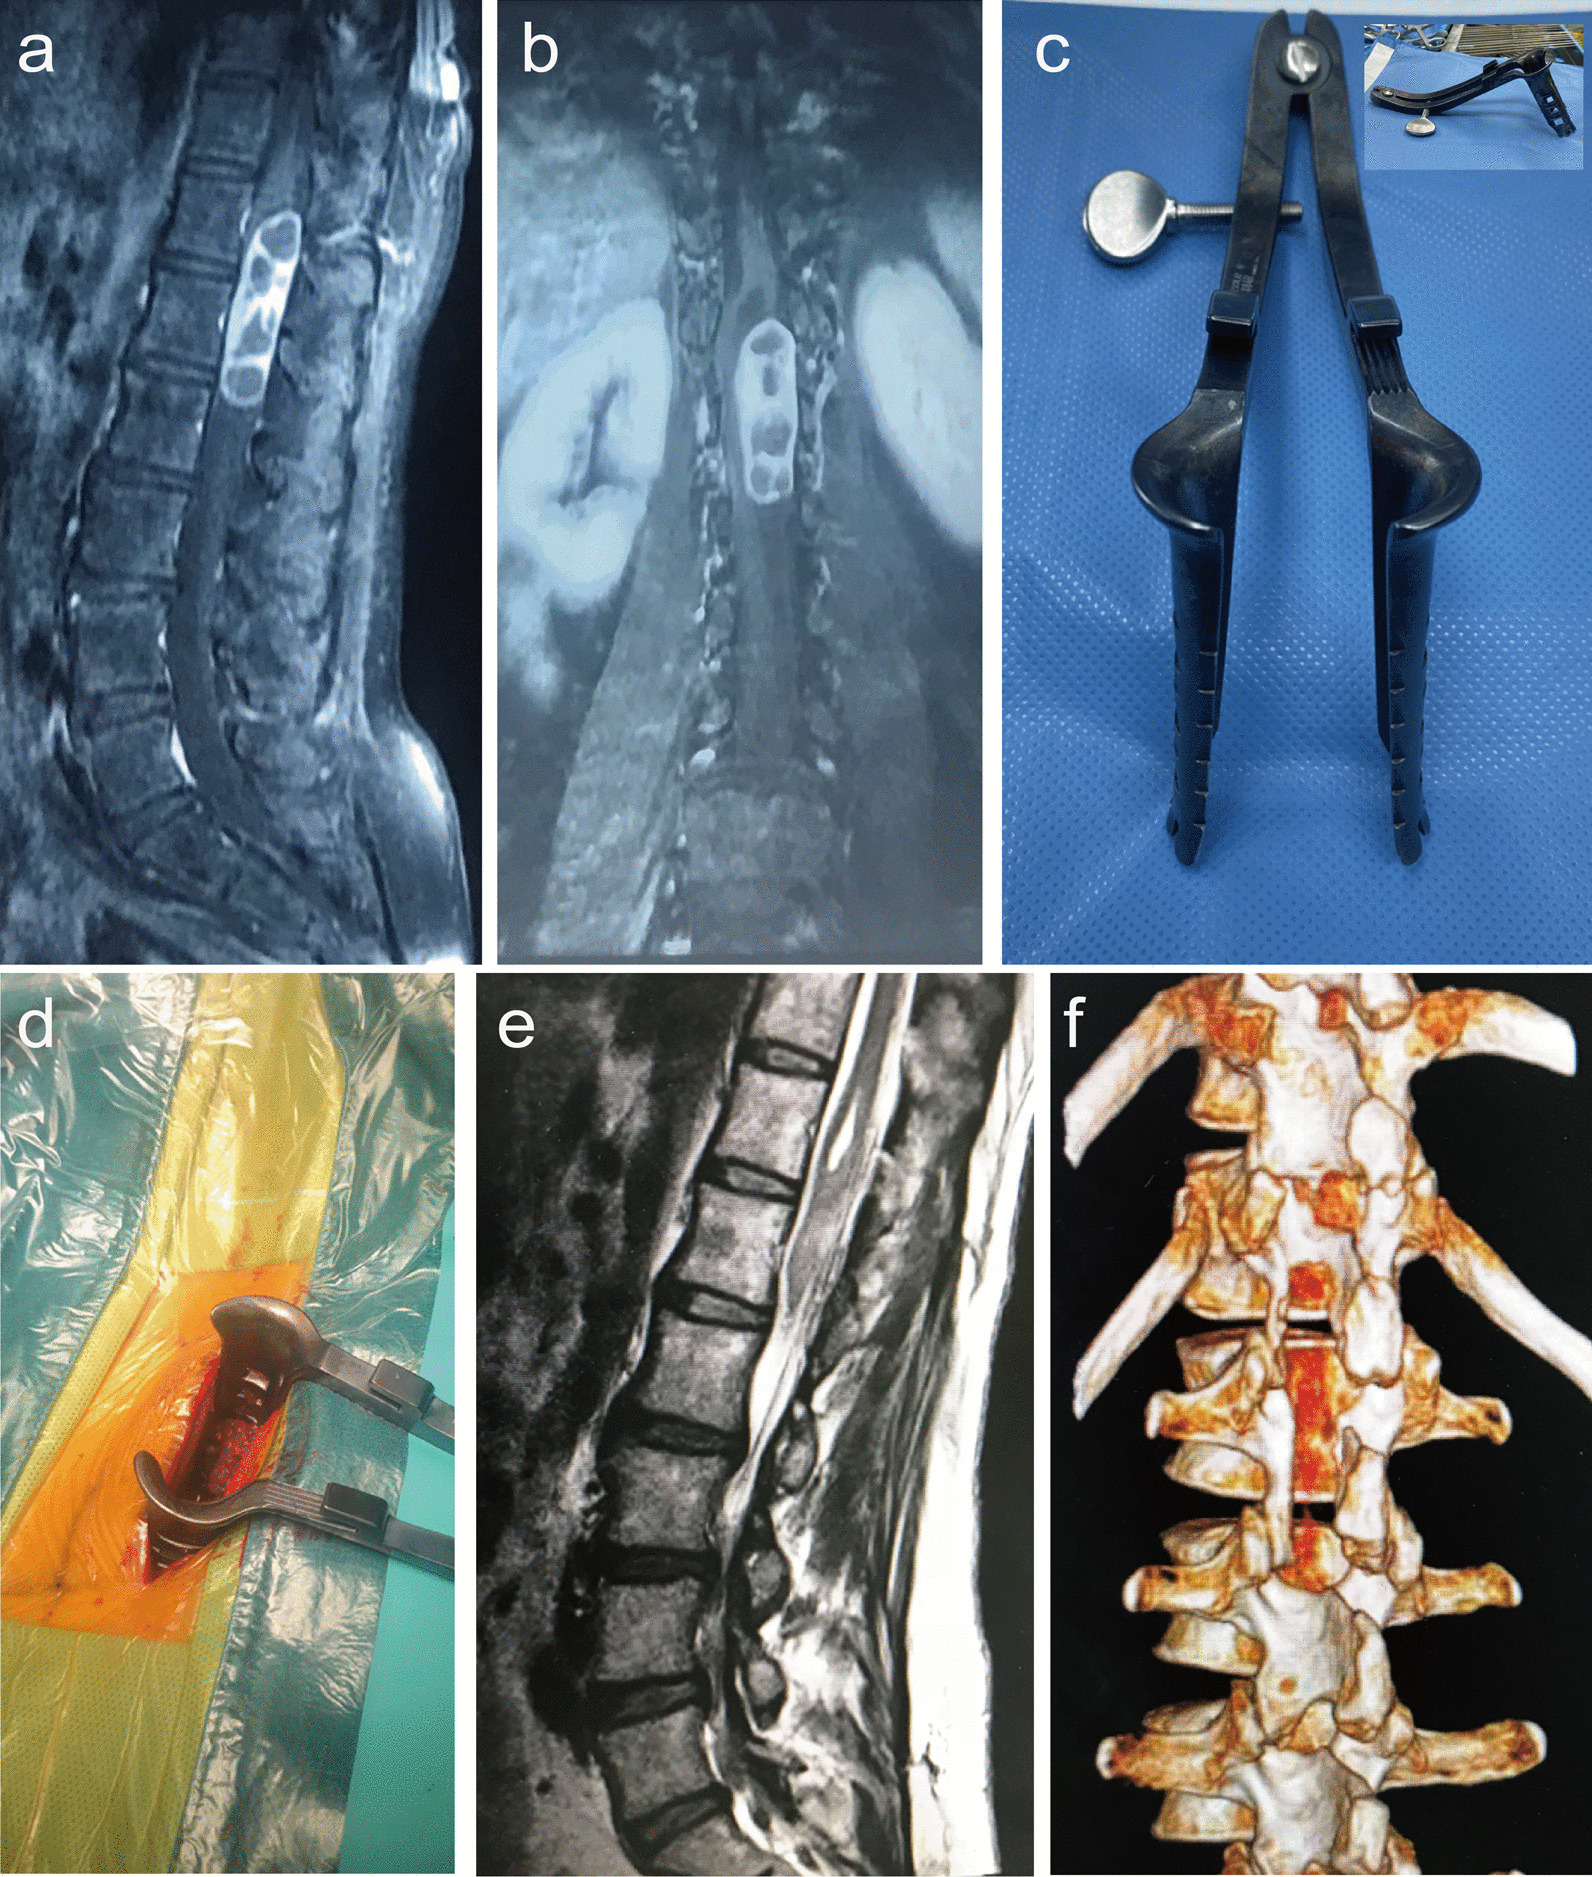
Typical case: a A 51-year-old woman presented with low back pain and numbness in her left lower limb for 1 year. Postoperative pathological diagnosis was intraspinal schwannoma. Physical examination showed hypoaesthesia in lateral side of the left leg and dorsum of feet. a, b MRI indicated a space-occupying lesion from T12 to L2 transpinal canal. c Real-object picture of the special retractor. d Posterior paramedian approach combined with inverted V-shaped surgical access. e, f Postoperative X-ray and three-dimensional reconstruction CT suggested that the tumor tissue was completely resected and intervertebral joint was damaged in a small range
